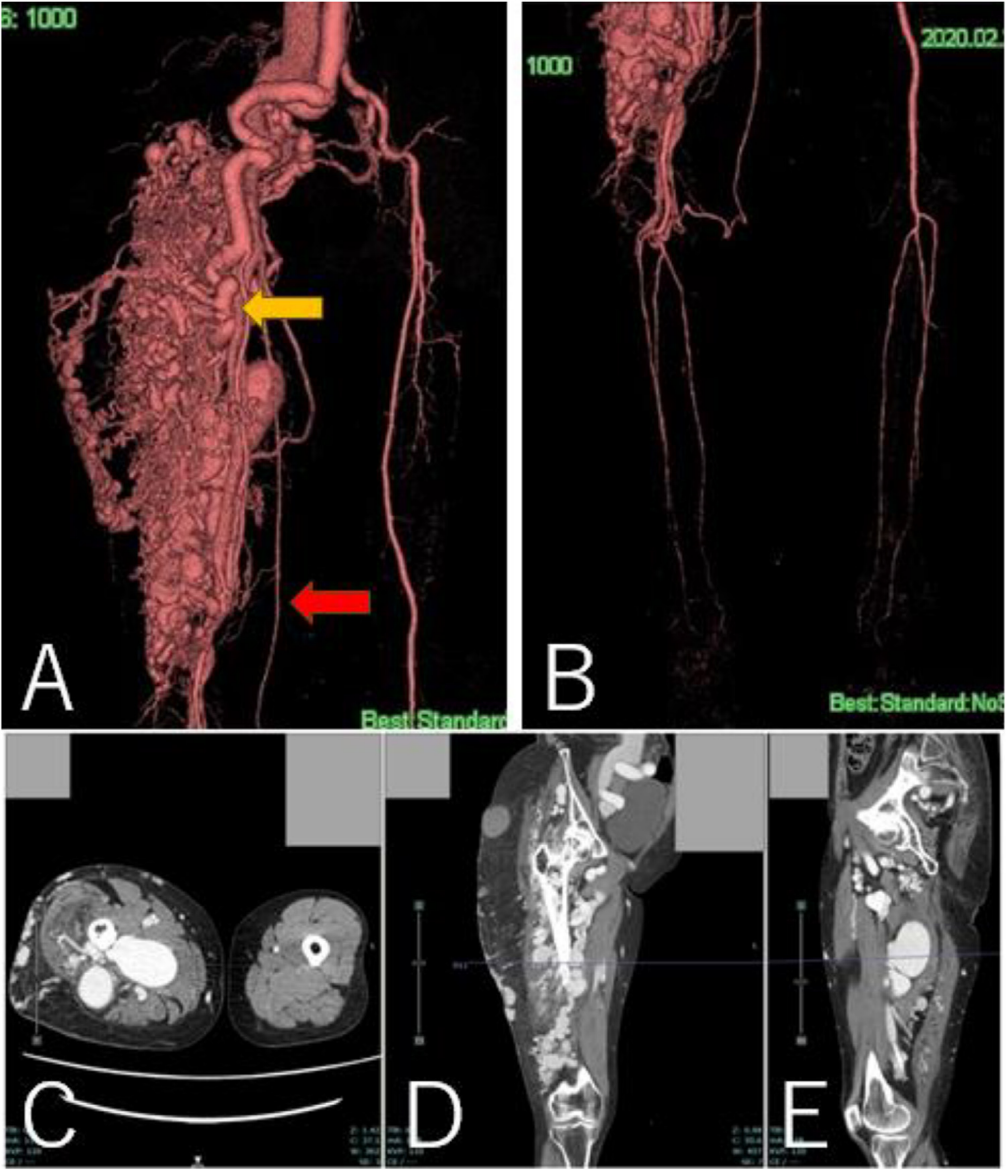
3D-CT angiography and multi-planar reconstruction of a giant arteriovenous malformation (AVM).(A) The feeding vessels included 4 branches from the right profunda femoris artery and the right superior and inferior gluteal arteries. One of the profunda femoris artery branches had a saccular arterial aneurysm (orange arrow, 20 × 17 × 27 mm). The right superficial femoral artery was hypoplastic and was not involved in the AVM (red arrow).(B) The giant AVM was located only in the right femoral region.(C) Axial view. (D) Coronal view. (E) Sagittal view.
